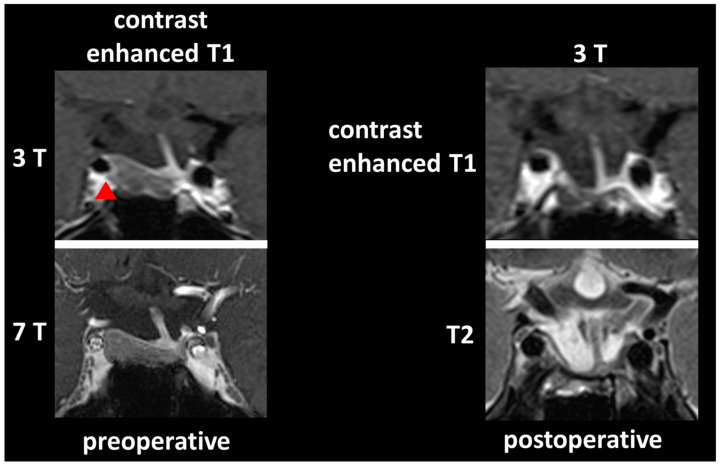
A 29-year-old Cushing patient with an ACTH secreting pituitary macroadenoma (contrast-spared, right-handed pituitary lesion): Knosp score was rated as “1” in preoperative 3 T MRI, which corresponds to a cavernous sinus infiltration not extending the median internal carotid artery (cavernous sinus infiltration marked with red arrowhead). In agreement with intraoperative findings, preoperative 7 T MRI showed no cavernous sinus infiltration with consequent Knosp grading “0”. Thus, complete tumor resection was achieved with consequent postoperative hormonal normalization. The 3 T MRI follow-up showed no residual tumor.
